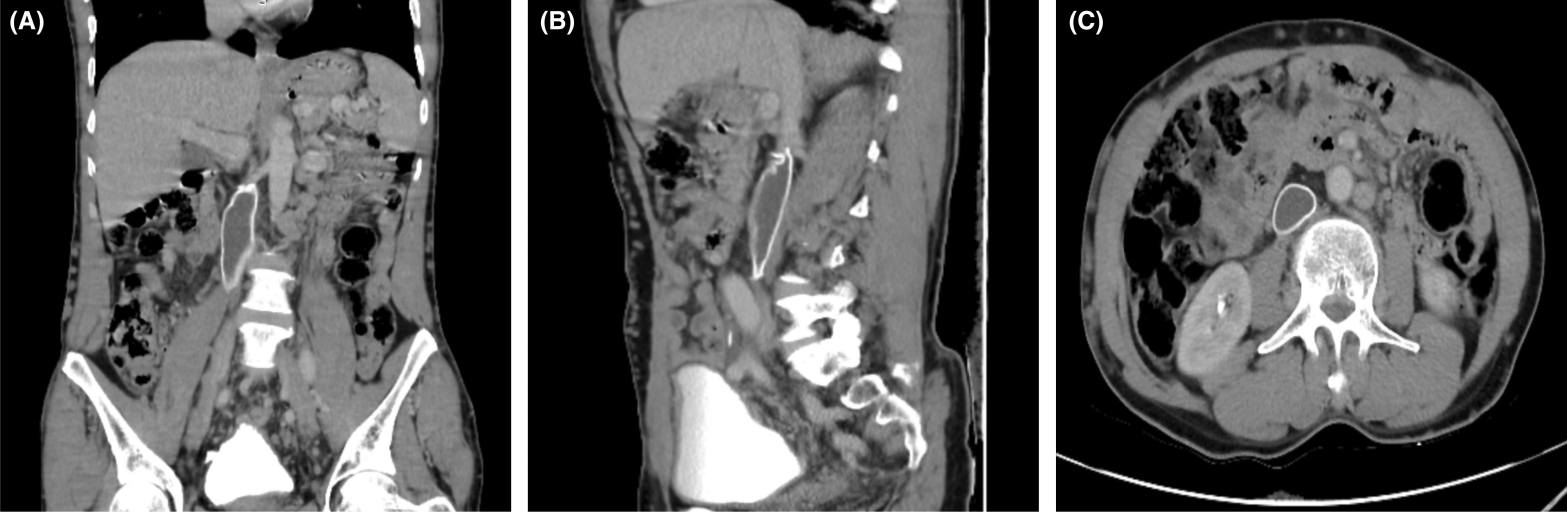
Postoperative abdominal Computed Tomography (CT) Scan at images (A–C) showing a functional surgical graft connecting the proximal inferior vena cava to the left common iliac vein (A, Coronal View; B, Sagittal View; C, Axial View).
